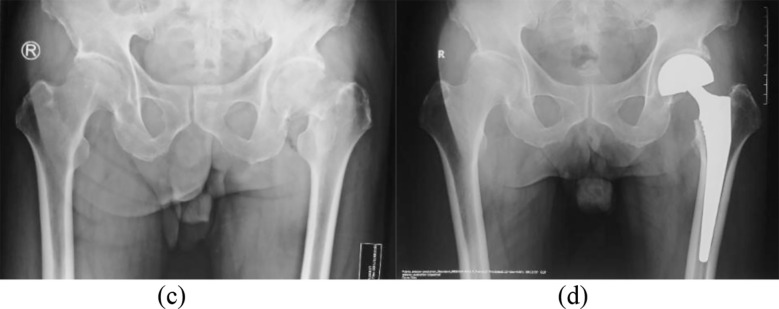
Preoperative and postoperative X-ray images BHA. *Note:* Male, 70 years old, underwent BHA for a left femoral neck fracture. (c) Preoperative X-ray; (d) Postoperative X-ray.
